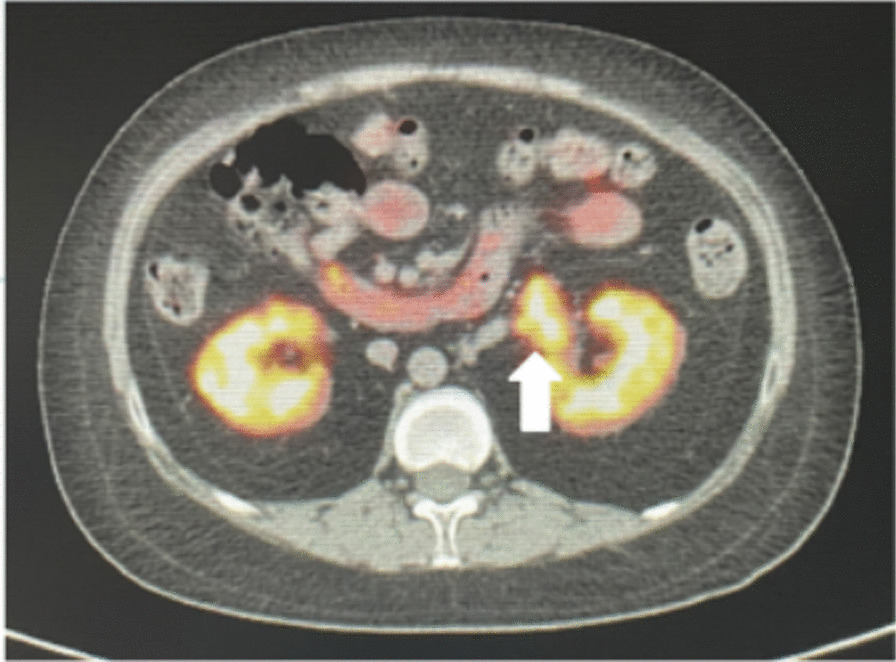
^68^Ga-DOTA-TATE-PET/CT images show a mass with significant enhancement in the left renal hilum (arrows)
